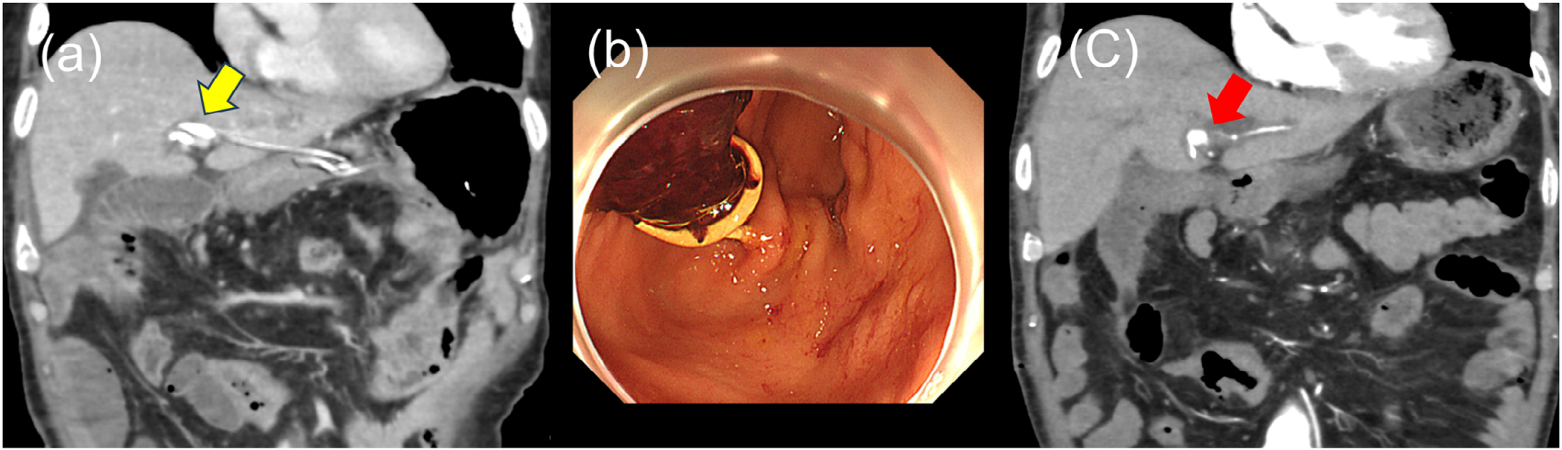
Images of hemobilia. (a) Computed tomography (CT) image of hemobilia at day 103. The bile duct shows patchy high density due to hemobilia. The tip of the hepaticogastrostomy (HGS) stent was coiled in a pigtail shape near B2+3 (yellow arrow). (b) Endoscopic findings. Blood clots were attached to the HGS stent, and blood flow from the stent's side hole was observed. (c) CT when the patient was in shock at day 106. The bile duct stent was removed. A pseudoaneurysm formed at the location of the HGS stent tip (red arrow).
